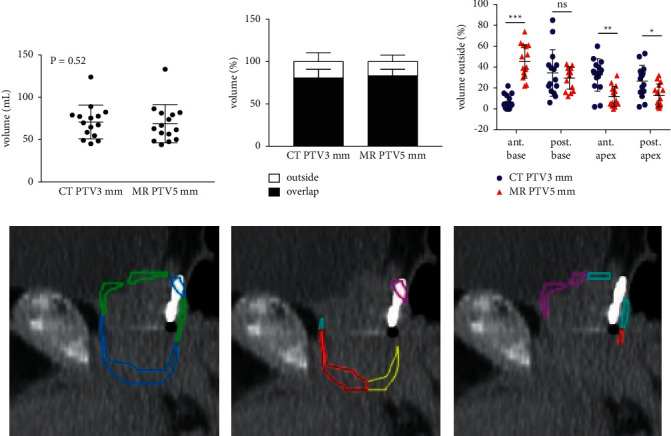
Discordance between CT and MR prostate contour by quadrant. Absolute volume (a) and percent overlap (b) of CT PTV3 mm and MR PTV5 mm are shown. (c) Percent volume by quadrant where the CT PTV3mm extends outside MR PTV5 mm is shown in blue, and percent volume by quadrant where the MR PTV5 mm extends outside the CT PTV3 mm is shown in red. Graphical representation of regions of discordance between CT PTV3 mm and MR PTV5 mm is shown in the panels. (d) Volume in green shows areas were the MR PTV5 mm extends outside the CT PTV3 mm, and volume in blue shows areas were the CT PTV3 mm extends outside the MR PTV5 mm. (e) Location (by quadrant) where CT PTV3 mm extends outside the MR PTV5 mm is shown. (f) Location (by quadrant) where MR PTV5 mm extends outside the CT PTV3 mm is shown. All panels show mean ± standard deviation. In panels A and C, individual values for all 15 subjects are shown. ^*∗*^*P* < 0.05, ^*∗∗*^*P* < 0.01, and ^*∗∗∗*^*P* < 0.001 by two-tailed paired Student's *t*-test or signed rank test.
